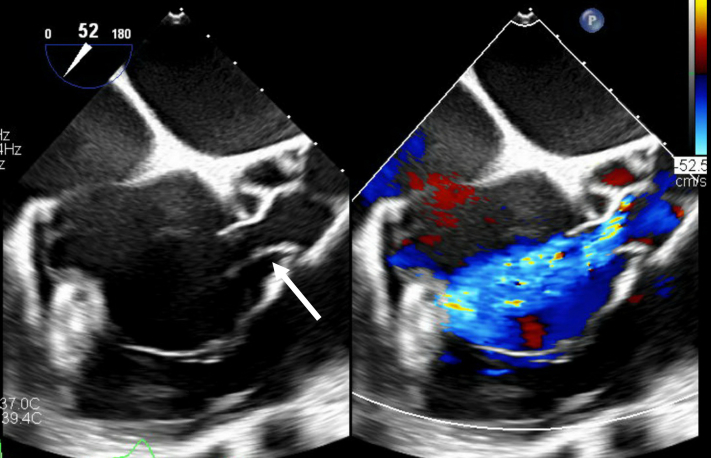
Initial Transesophageal Echocardiogram Showing Aorto-Atrial Fistula Open arrow indicates the aorto-atrial fistula.
